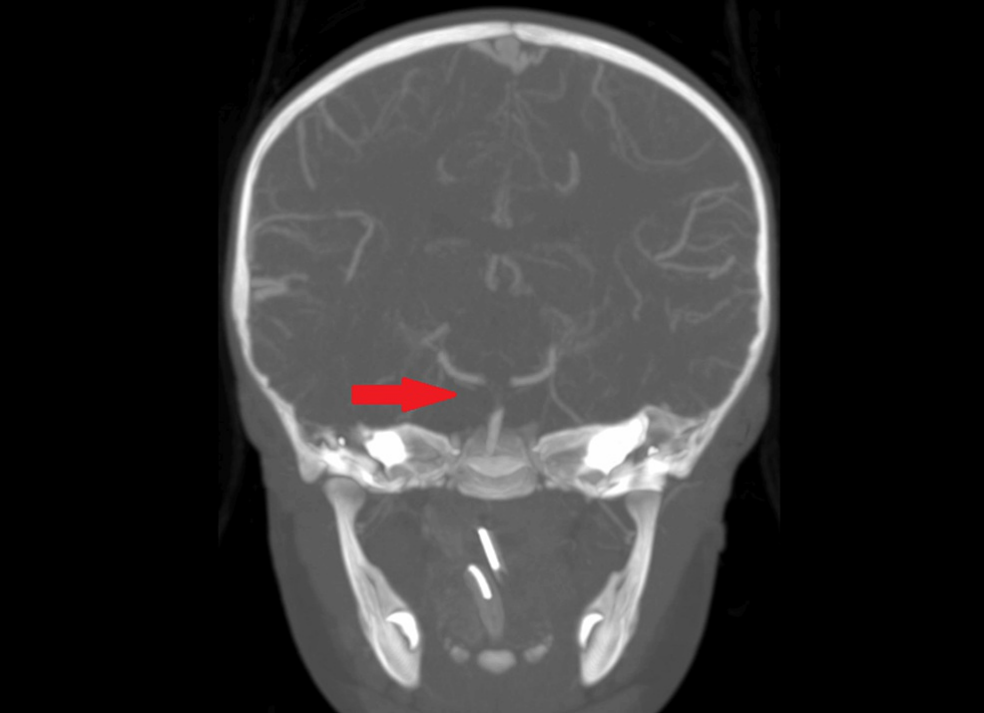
Coronal maximum intensity projection computed tomography angiography demonstrating distal basilar occlusion.
